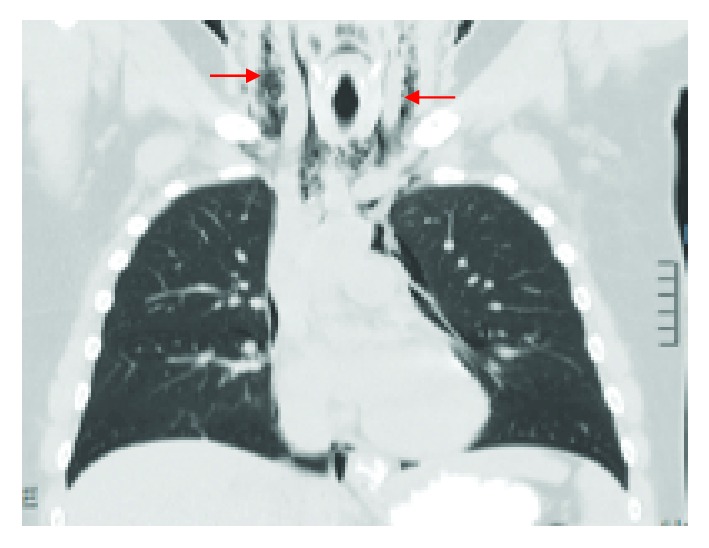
CT chest demonstrating extension of air in the cervical region.
